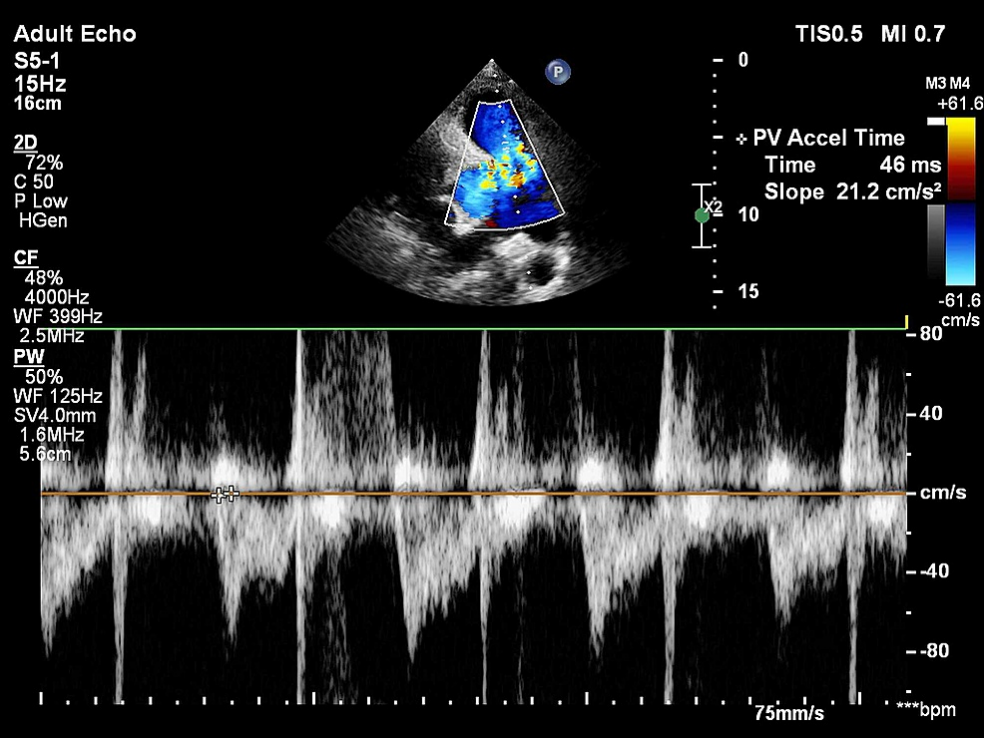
Pulsed wave (PW) Doppler showing a reduced pulmonary acceleration time (PAT) and early systolic notching (ESN)
